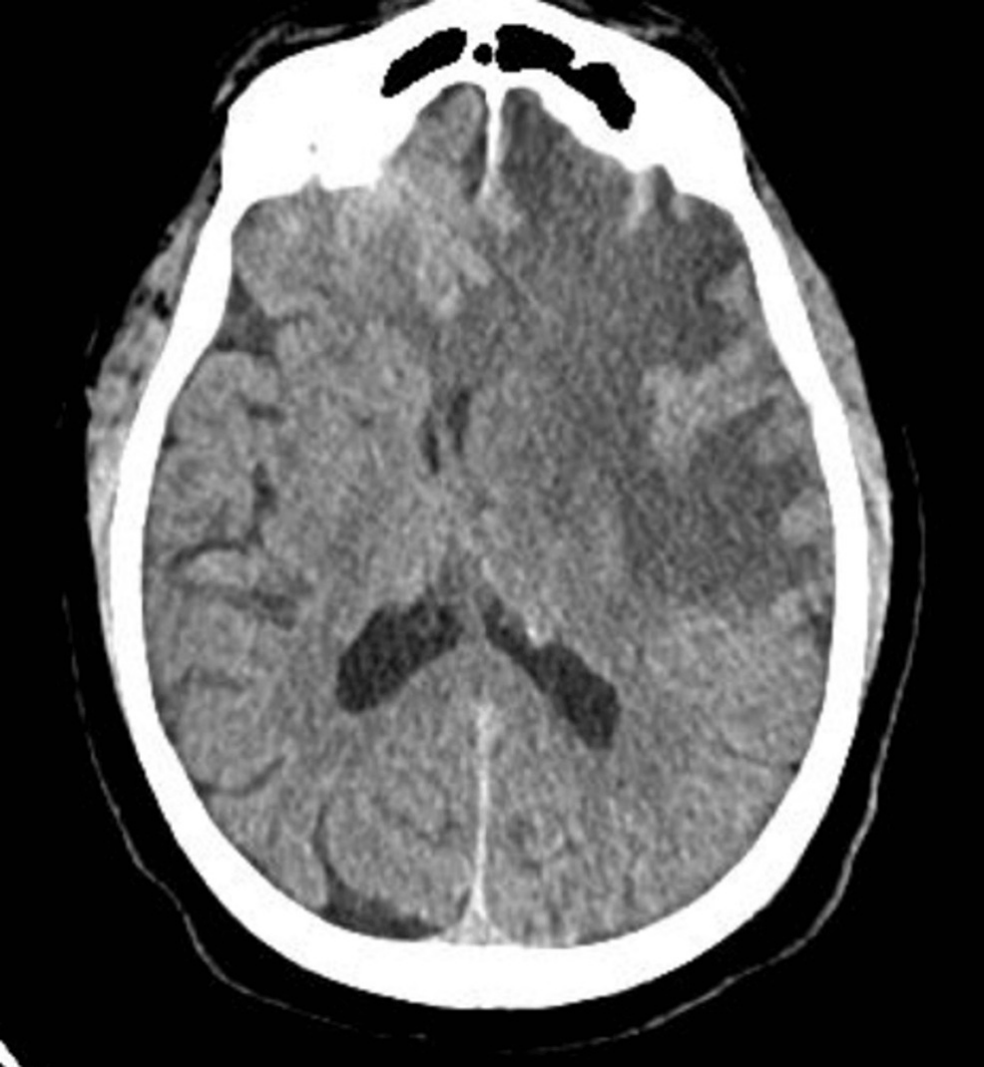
Head CT was done on presentation, showing hypo attenuated area and mass effect
